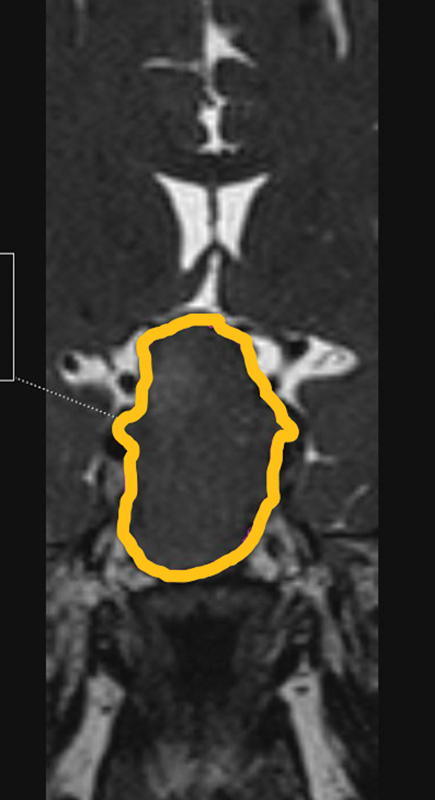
Preoperative tumor volume measured using 3D magnetic resonance imaging (yellow outline).
